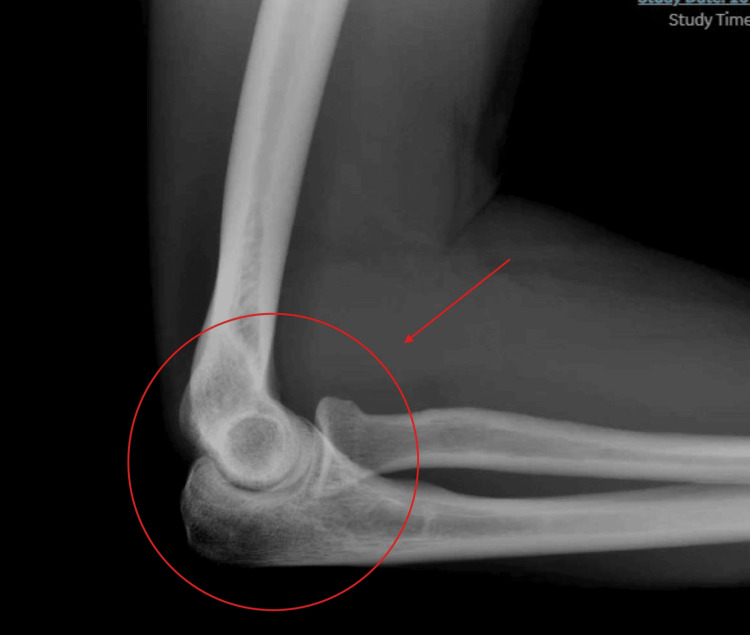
Lateral radiograph of the right elbow demonstrating normal osseous alignment The highlighted section demonstrates normal osseous alignment of the bone.
